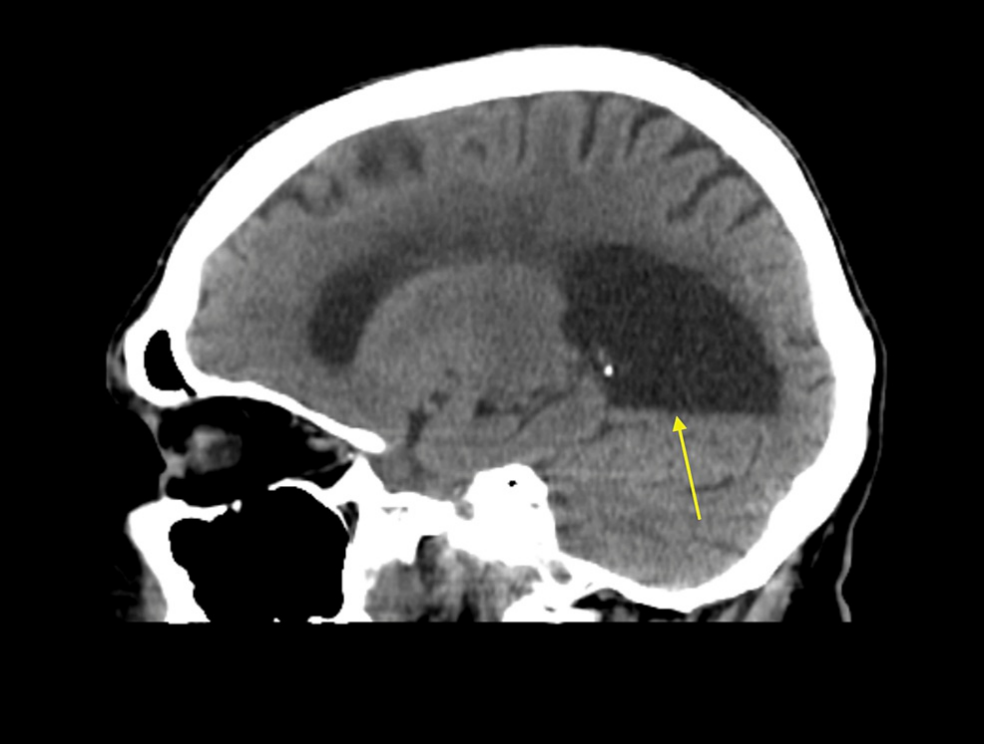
CT scan of the head: sagittal view. Asymmetric dilatation of the right occipital horn due to agenesis of corpus callosum resulting in colpocephaly.
